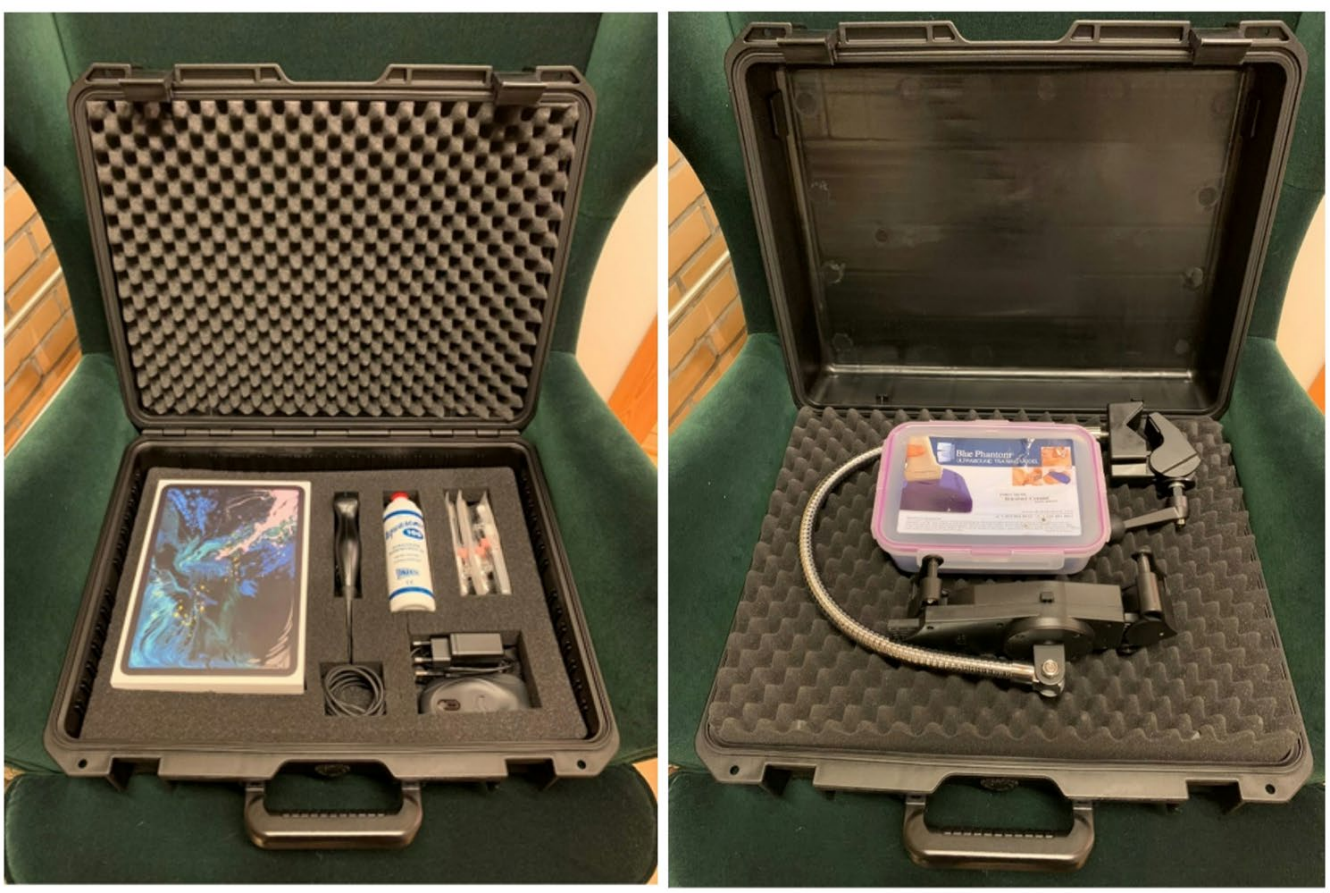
Suitcase with ultrasound equipment sent to all the students in the remote group
